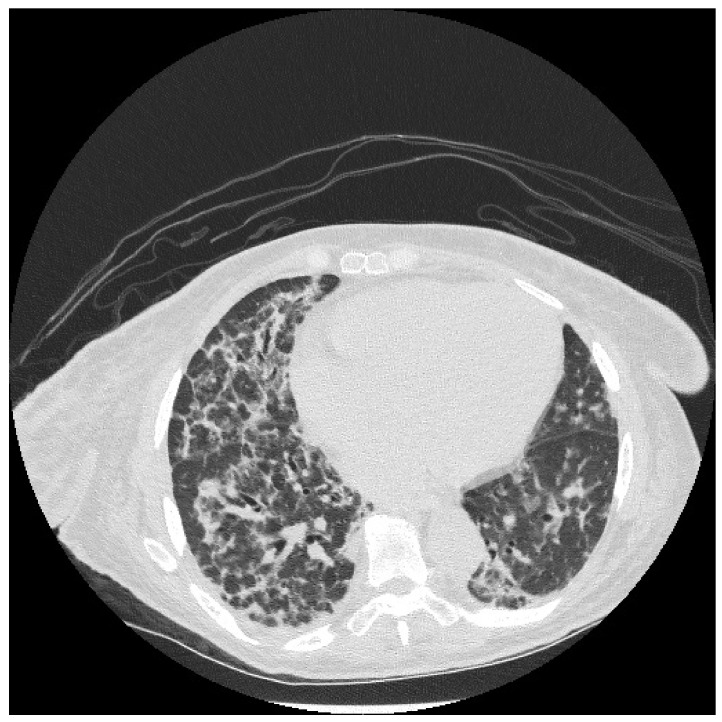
CT of the chest in the lower lung fields with organizing pneumonia with interstitial reticulations, ground glass opacities, peribronchial inflammation, and consolidation.
